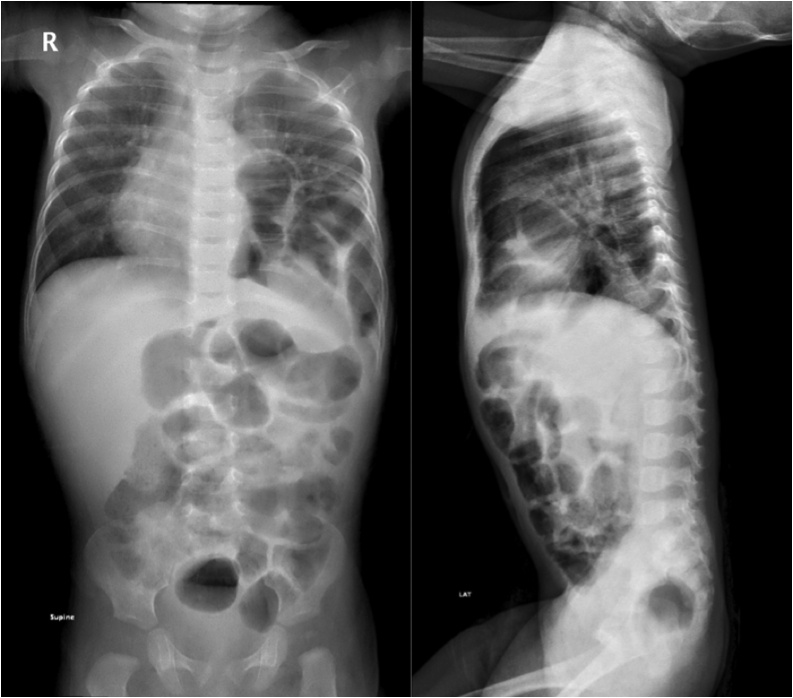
Anteroposterior and lateral chest x-ray showing bowel segments in the left hemithorax demonstrating recurrence of CDH.
